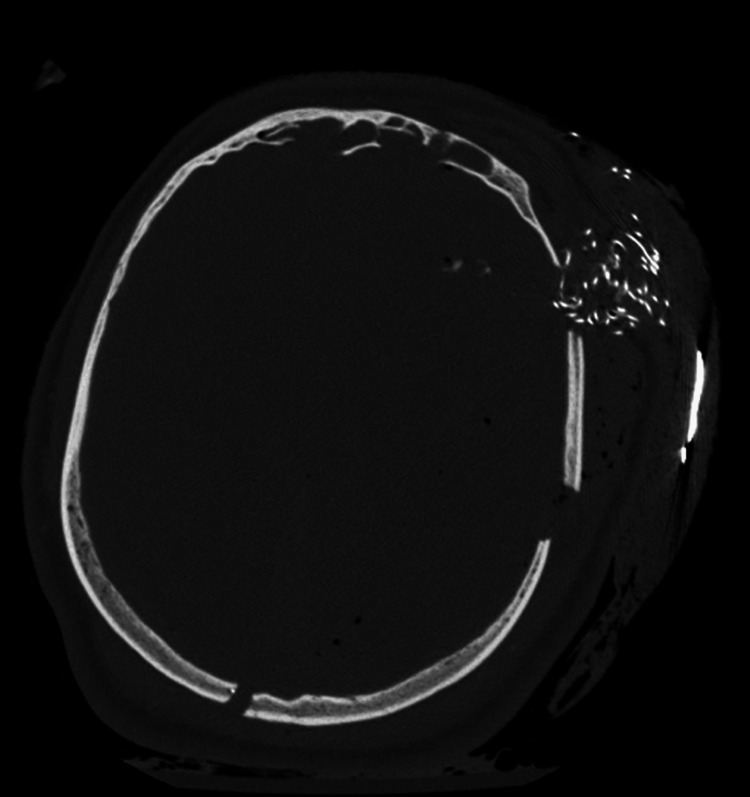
CT scan of the skull taken in an Axial (horizontal) view with BIPP packing incorporating radiopaque marker strips CT: computed tomography, BIPP: bismuth iodoform paraffin paste
